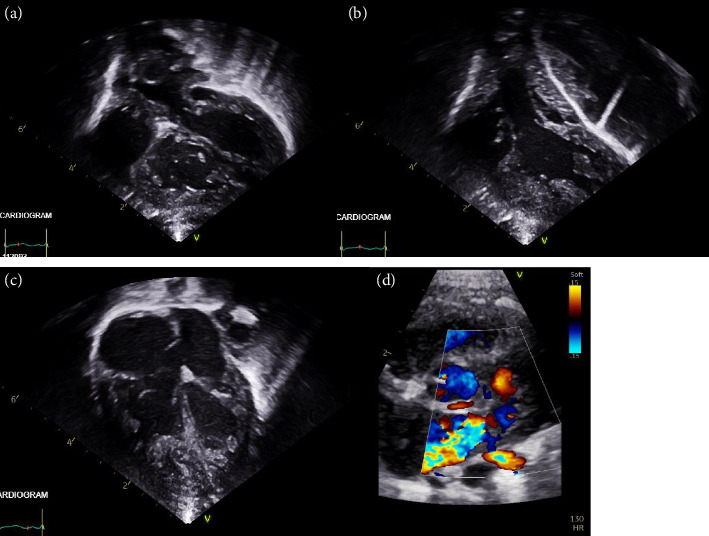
Postnatal echo: (a) subcostal view demonstrating pulmonary artery arising from the left ventricle, (b) subcostal view demonstrating aorta arising from the right ventricle, (c) apical 4-chamber view demonstrating large interatrial communication and hypertrabeculations of both ventricles, and (d) parasternal short-axis view demonstrating the circumflex artery running posteriorly to the pulmonary artery.
